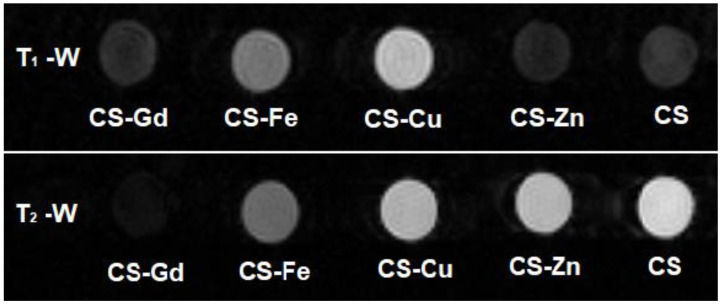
T_1_-weighted (top) and T_2_-weighted (bottom) MR images of CS–metal and CS microgel water dispersions obtained with 0.5 T MRI scanner. (T_1_-weighted image IR sequence parameters: TR = 5000 ms, TE = 20 ms, TI = 1000 ms; T_2_-weighted image SE sequence parameters: TR = 5000 ms, TE = 26 ms).
